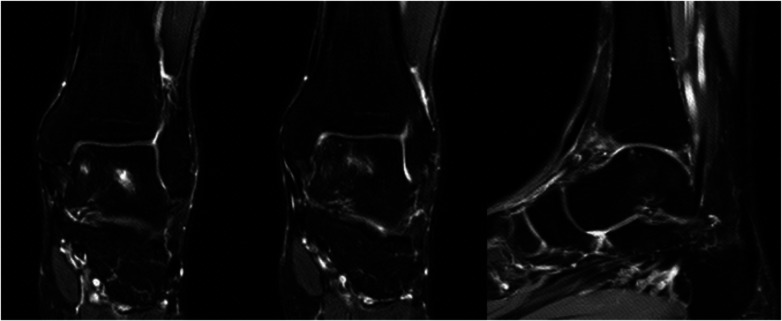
MRI review showed that the talus cartilage was repaired satisfactorily and the edema area was significantly reduced 5 months after surgery.
